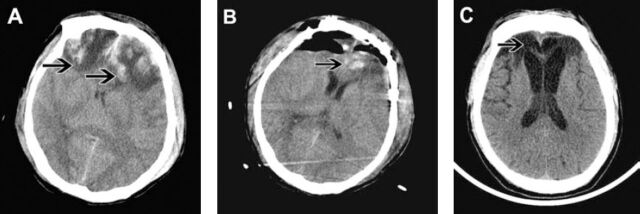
A 48-year-old male with bilateral frontal lobe contusions and hematomas that were removed through a coronal incision. Non-contrast axial head computed tomography scans showing: A) pre-operative scan after injury; B) post-operation; C) follow-up with good recovery.
